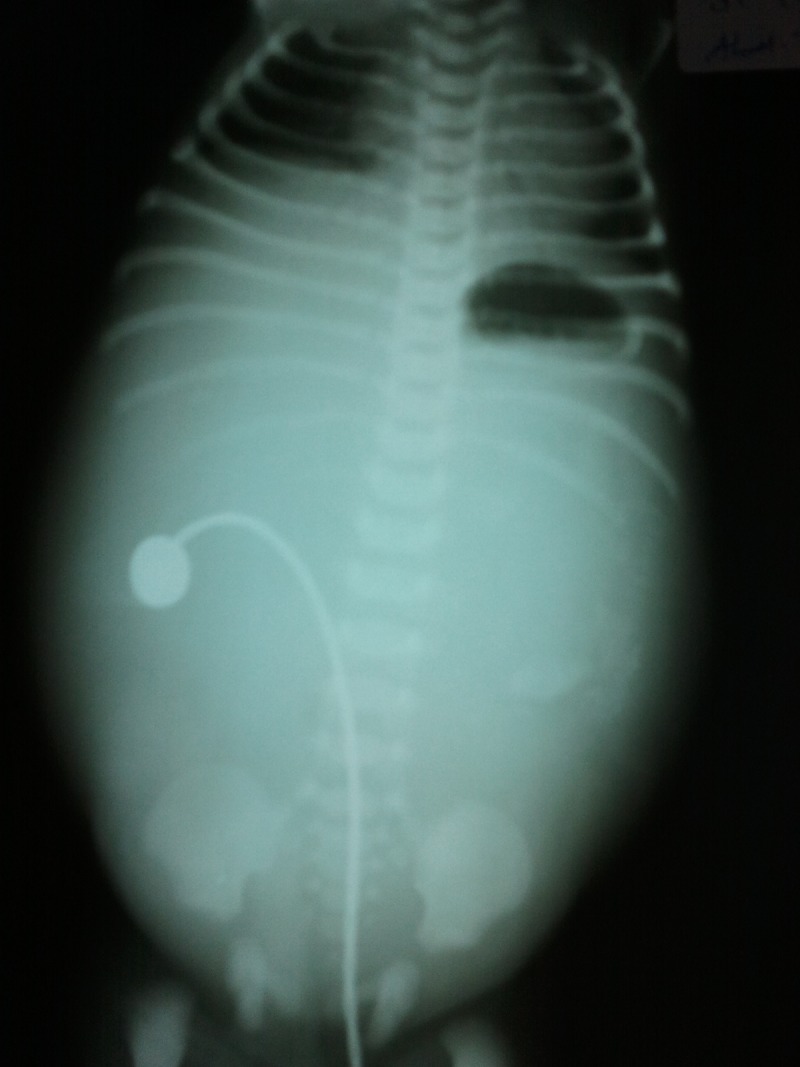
Figure 1: Radiograph showing single air bubble with calcifications in left flank
